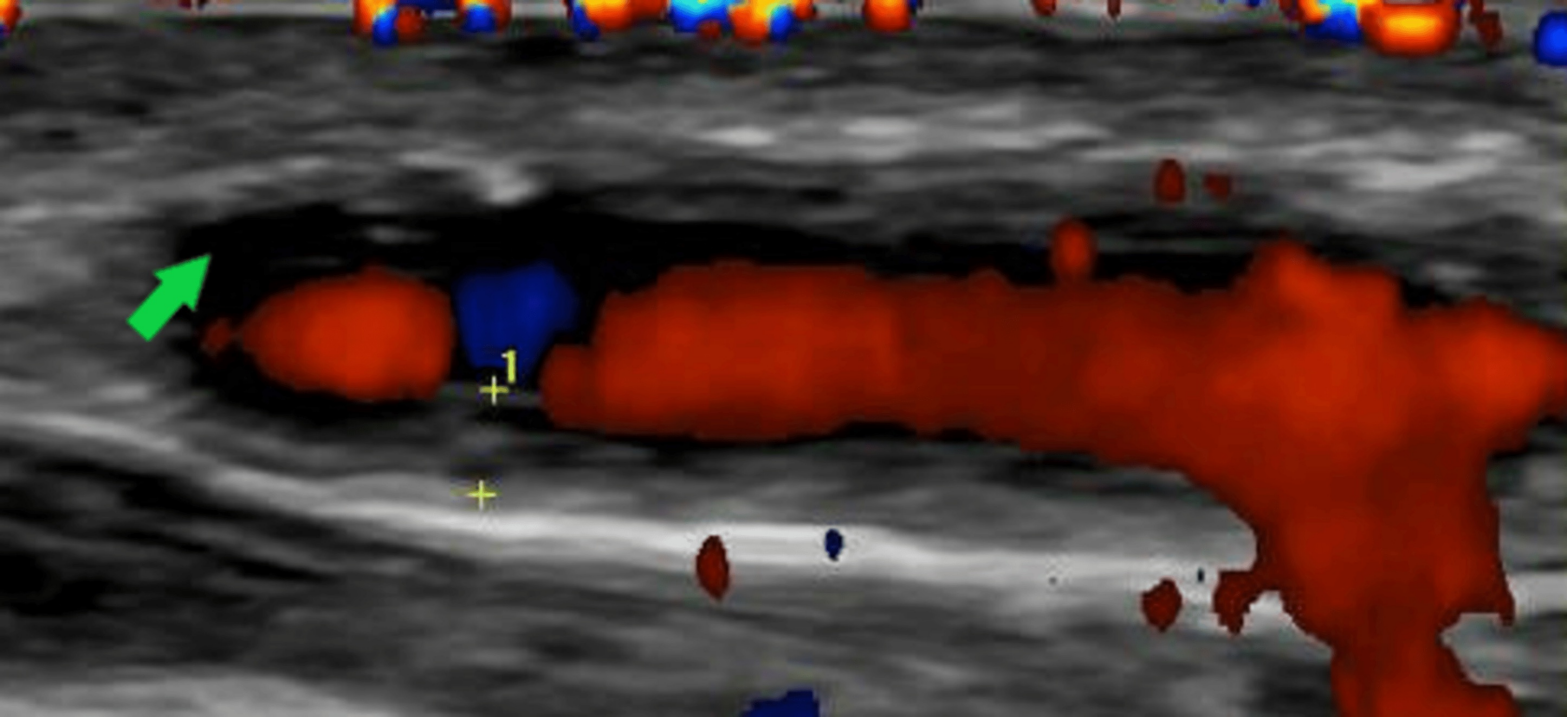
Colour Doppler ultrasound showing a longitudinal view of the frontal branch of the left temporal artery which demonstrates a thickened arterial wall with a narrowed lumen due to the inflamed and swollen arterial walls
